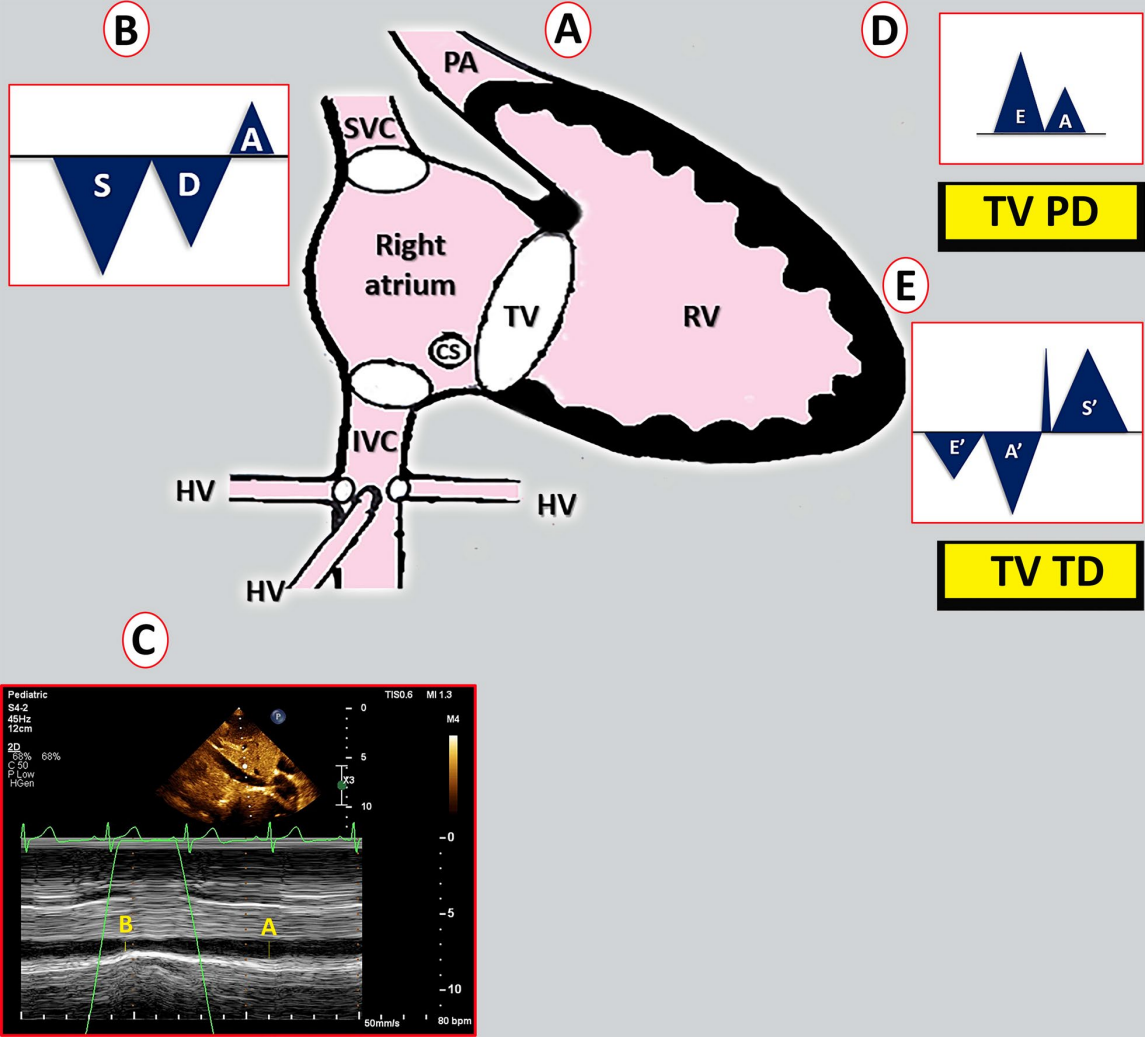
A The heart and the connections of the right atrium to the surrounding vessels. B The Doppler profile of the inferior vena cava, superior vena cava, and hepatic vein. C The measurement of maximal and minimal diameters of the inferior vena cava. D The pulse-wave Doppler of the tricuspid valve flow. E The tissue Doppler of the lateral annulus of the tricuspid valve (*IVC* inferior vena cava, *SVC* superior vena cava, *HV* hepatic vein, *TV* tricuspid valve, *PW* pulse Doppler, *TD* tissue Doppler)
